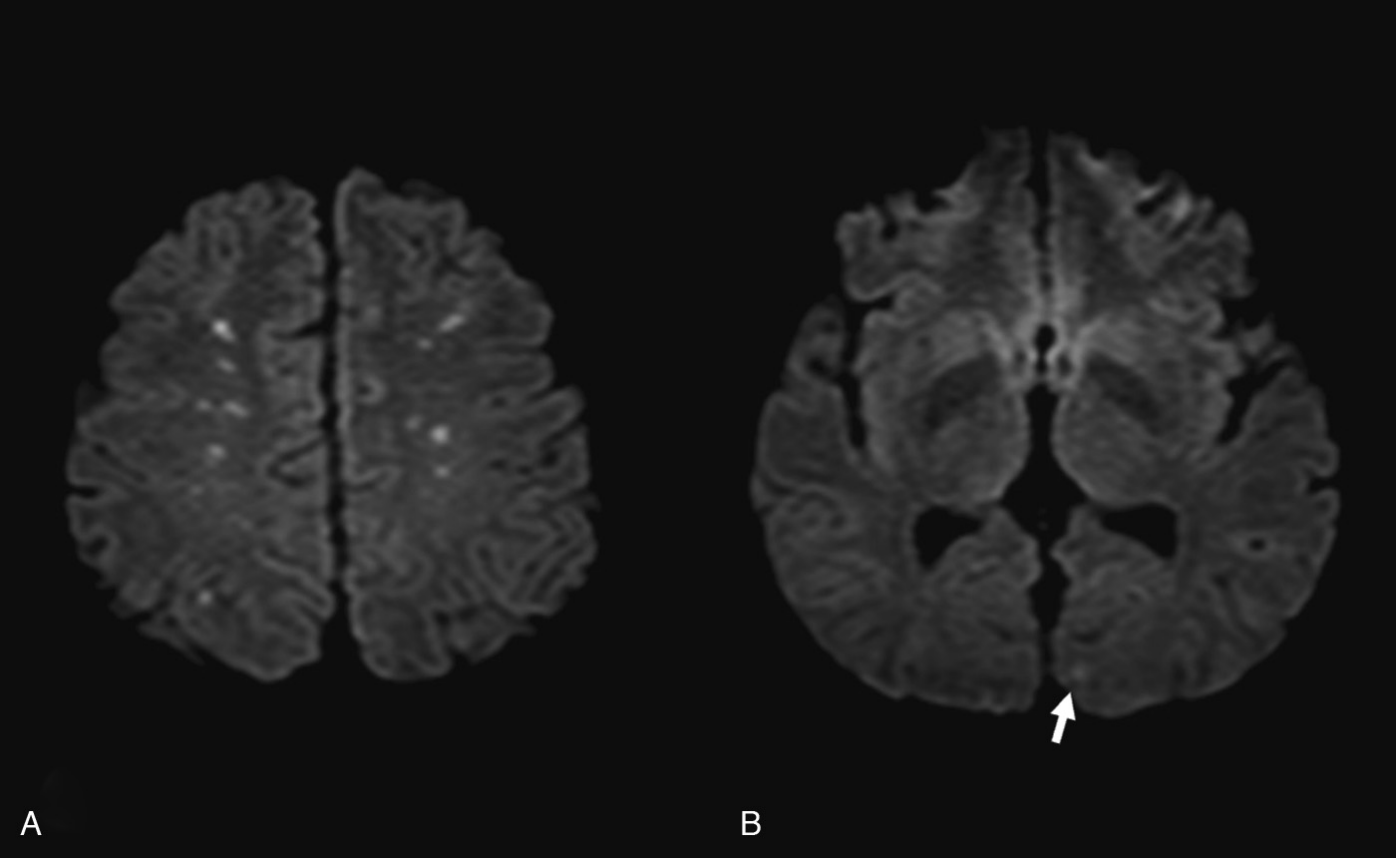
Diffusion weighted imaging series of brain magnetic resonance imaging (MRI) showed multiple disseminated small infarctions in the white matter of the (A) bilateral cerebral hemispheres and (B) left occipital region (arrow).
